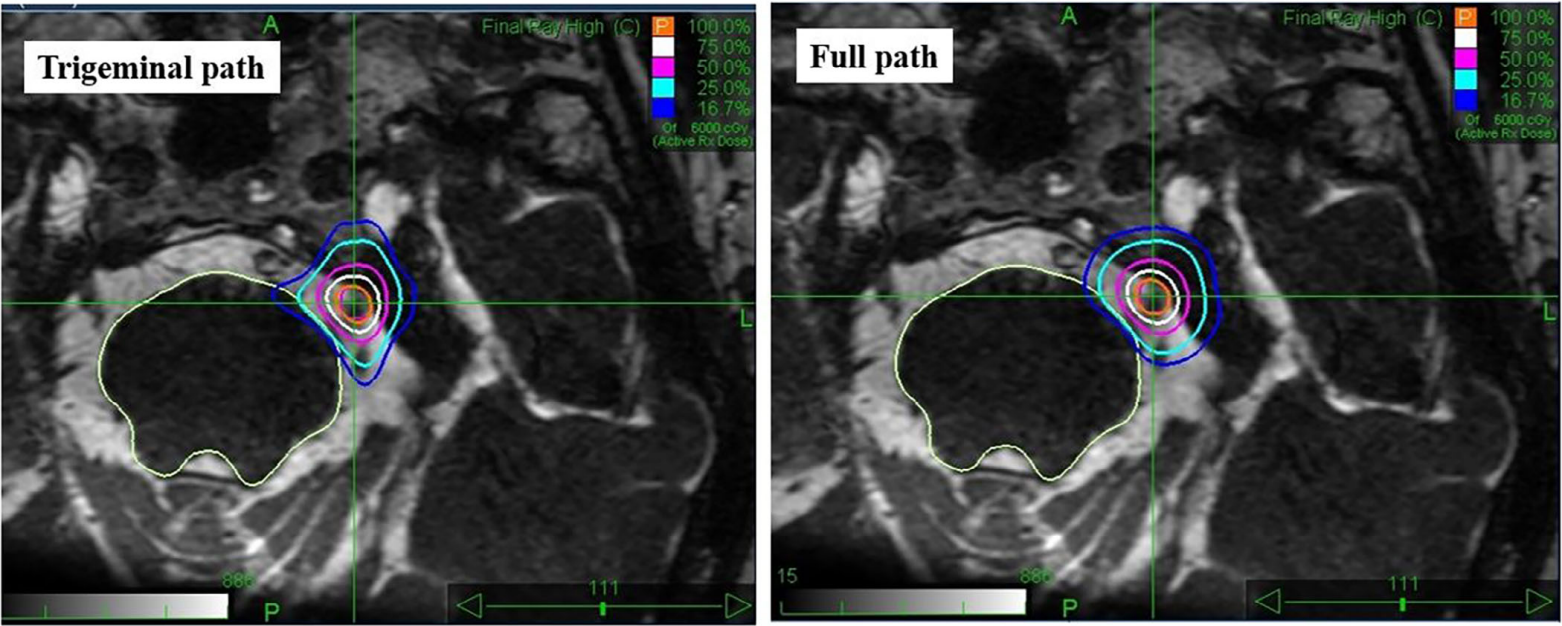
The dose distributions planned using the trigeminal path (gradient index = 5.4) and full path (gradient index = 5.9) for the same patient on a SPACE T2‐weighted MR image.
